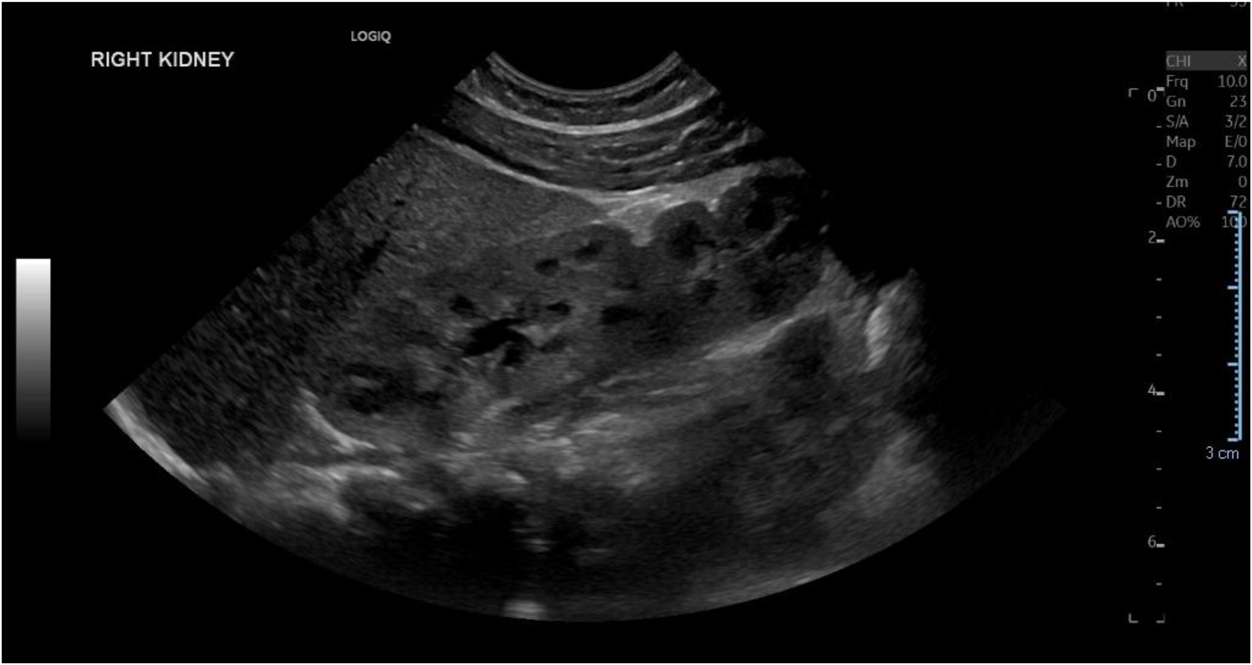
Ultrasound of the right kidney demonstrating CFRE with 2 distinct moieties with preserved corticomedullary differentiation and mild hydronephrosis (July 8, 2025).
